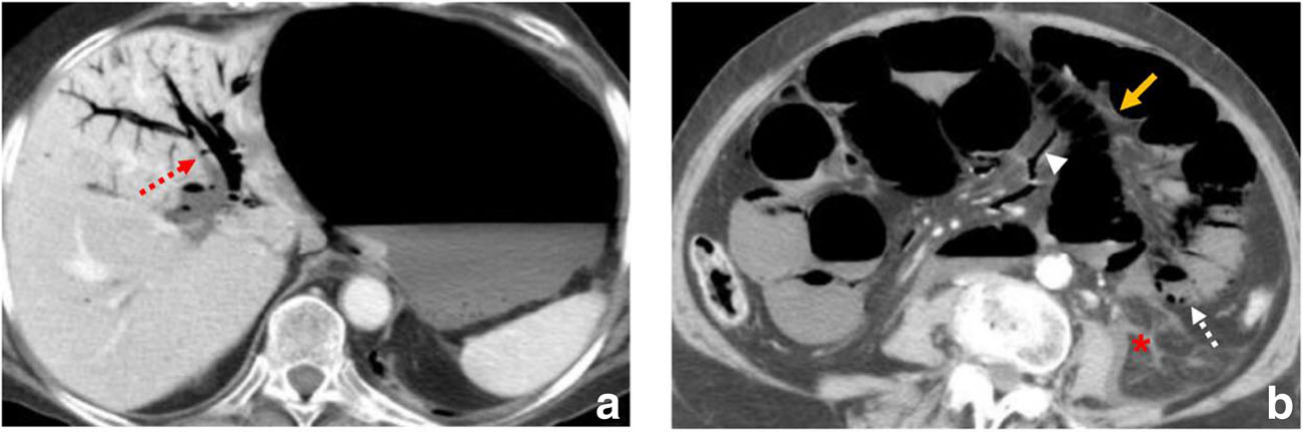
Contrast-enhanced CT image of the abdomen in an 82-year-old male with embolism of the superior mesenteric artery. (a) Contrast-enhanced axial CT shows pronounced intrahepatic portal venous gas (branching hypoattenuating areas) extending into the periphery of the left liver lobe (red arrow). (b) Contrast-enhanced axial CT scan shows dilated and gas-filled loops with an extreme thinning of the bowel wall, a “paper thin wall”, due to transmural small-bowel infarction (orange arrow). Pneumatosis (white arrow) is also seen; fat stranding (*) and gas in mesenteric veins (white arrowhead)
